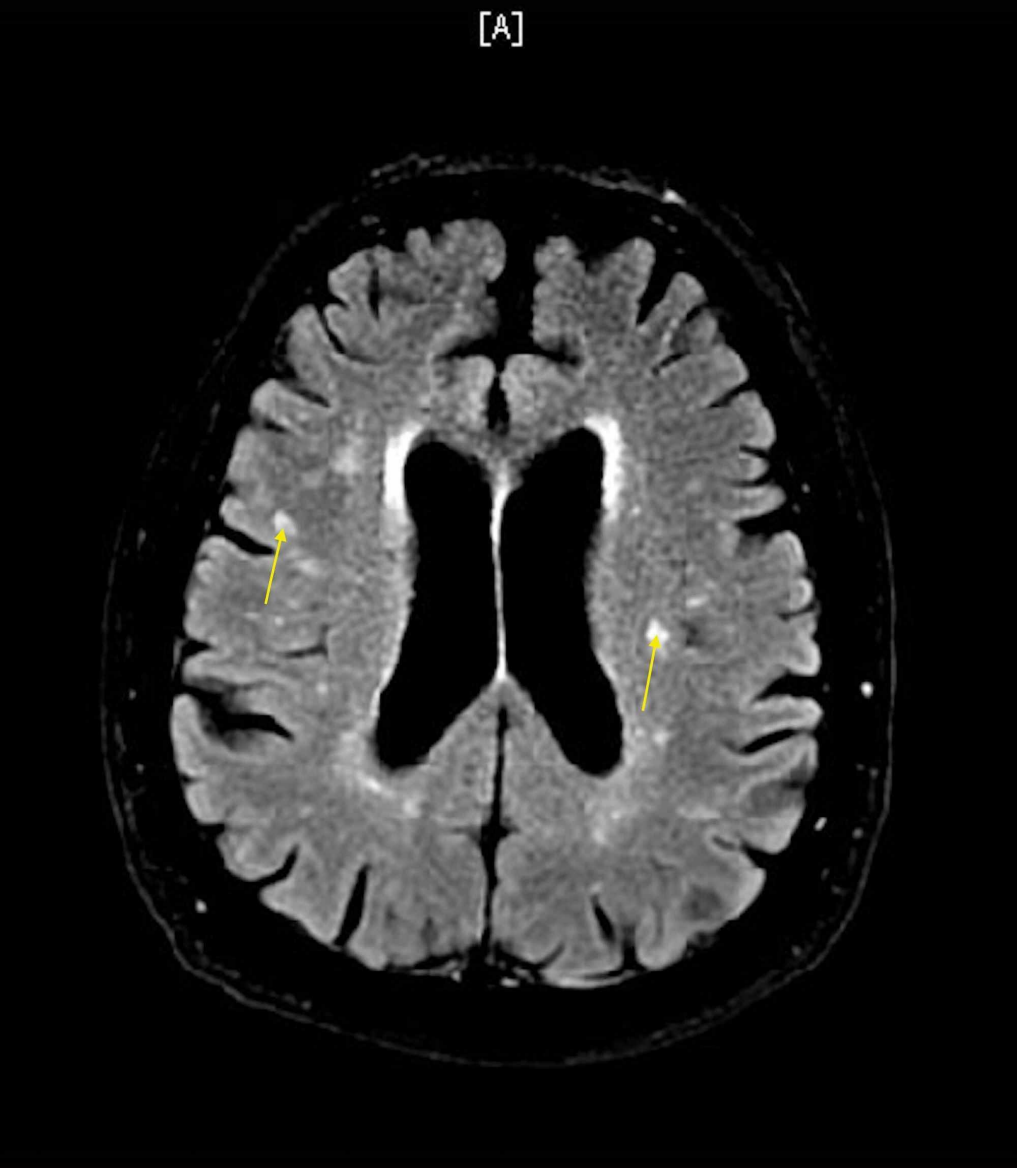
MRI brain without contrast showing white matter hyperintensities, consistent with chronic white matter ischemic changes
